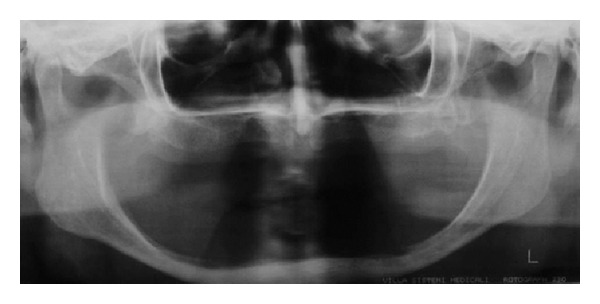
Panoramic radiograph showing severe resorption of mandibular alveolar ridge.
